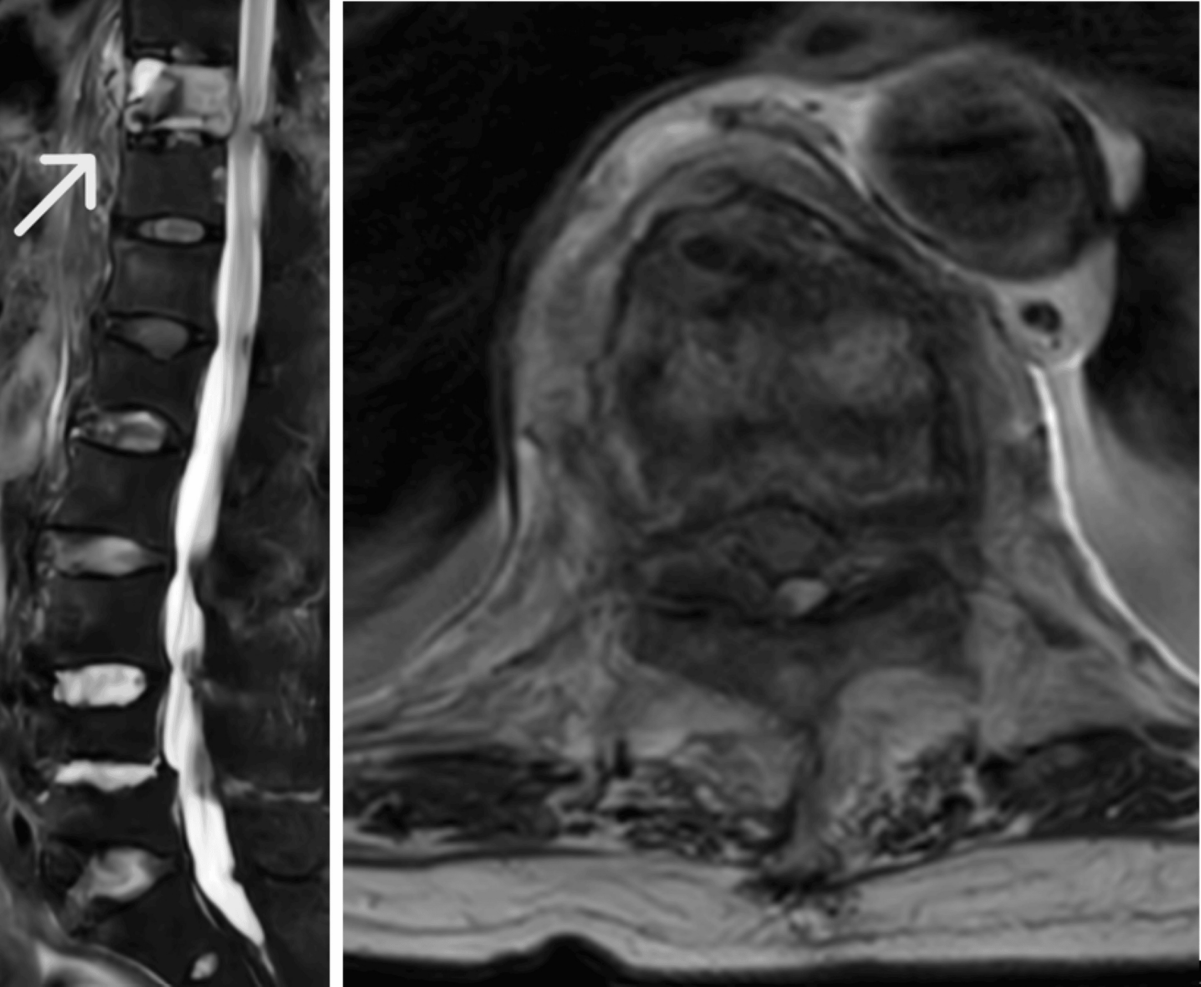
Magnetic resonance imaging (MRI) at diffuse idiopathic skeletal hyperostosis (DISH) spine diagnosis The sagittal STIR MRI showed the signal changes of the Th10 vertebral body and posterior ligaments suggesting acute fracture. The T2 axial view showed cord compression at T10/11 level and hematoma formation around the T10 vertebral body.
